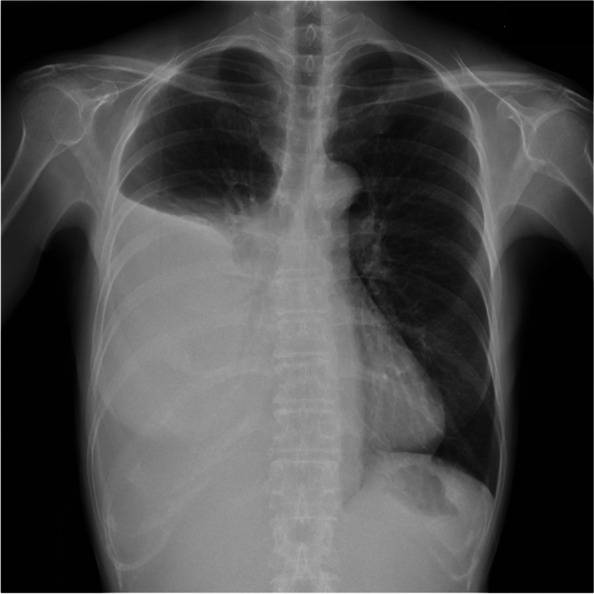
Chest radiography revealed a right massive pleural effusion
